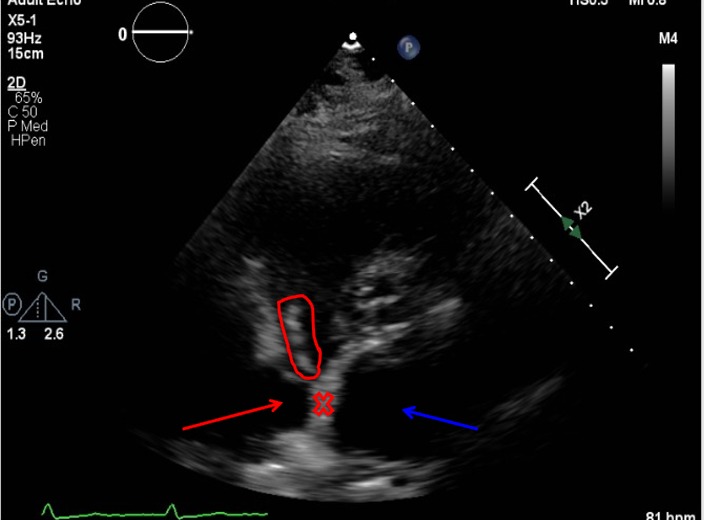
Two-chambered 2D echocardiogram showing the vegetation on the right side of the interatrial septum Red circle = vegetation, red x = interatrial septum, red arrow = right atrium, blue arrow = left atrium 2D: two-dimensional
